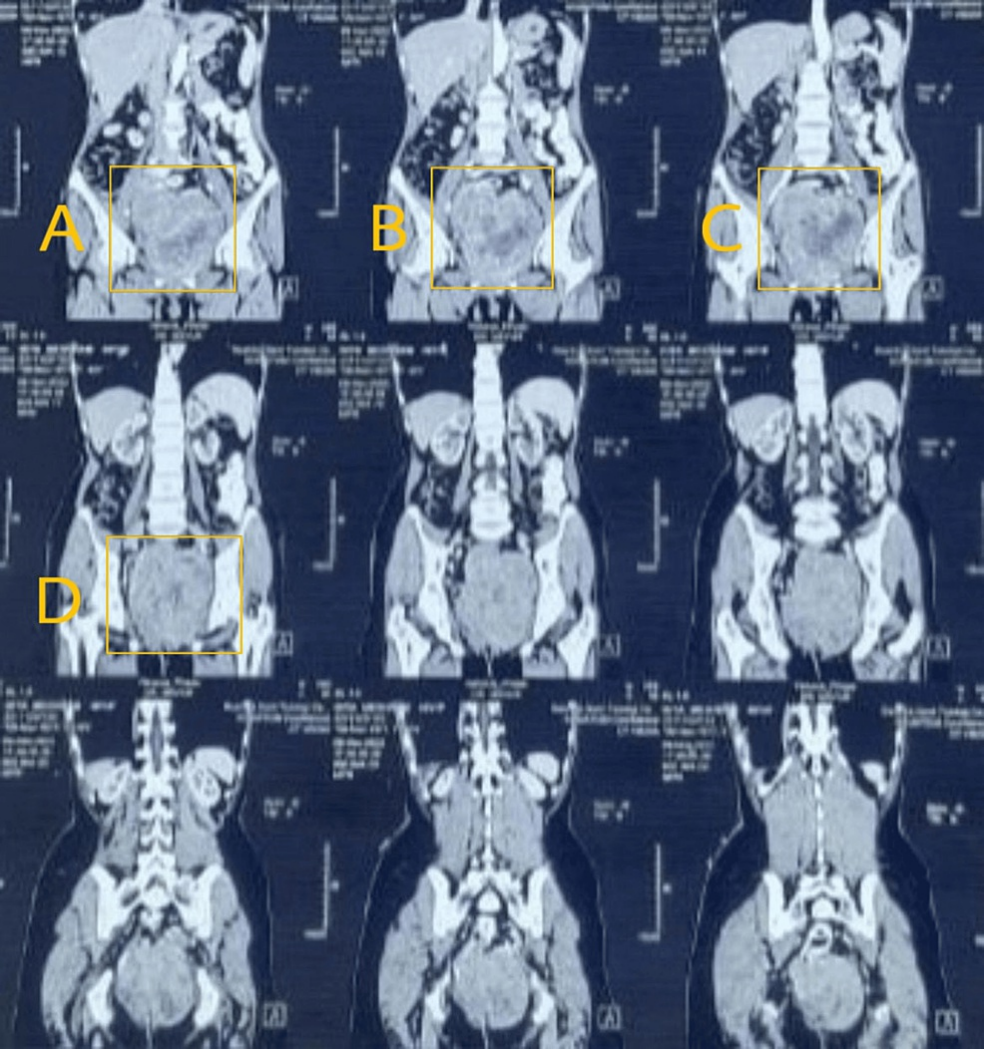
MRI showing GIST Highlighted areas of A-D showing the tumors in different panes GIST: gastrointestinal stromal tumors; MRI: magnetic resonance imaging
